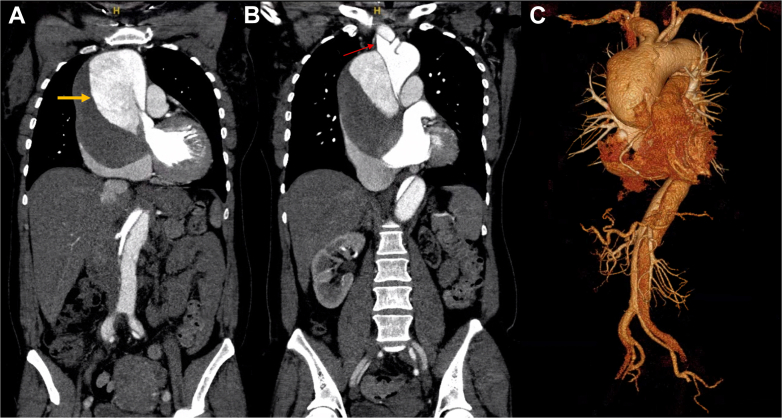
Preoperative Computed Tomography Angiography Demonstrating Chronic Stanford Type A Aortic Dissection With Thrombosed False Lumen (A) Coronal computed tomography angiography (CTA) demonstrating a chronic type A dissection with aneurysmal dilation of the ascending aorta and thrombosed false lumen (arrow). (B) Coronal CTA slice showing arch vessels arising from the true lumen (arrow). (C) Three-dimensional reconstruction of the thoracic and abdominal aorta demonstrating the extent of the dissection flap and aneurysmal dilation of the ascending aorta with distal extension into the abdominal aorta.
